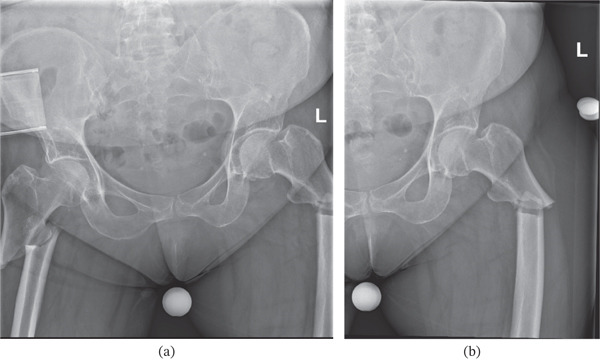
(a) Initial anteroposterior radiograph showing complete bilateral subtrochanteric femoral fractures consistent with atypical femoral fractures (AFFs) and (b) additional isolated anteroposterior radiograph of the left femur for a definitive view of bilateral fractures.
